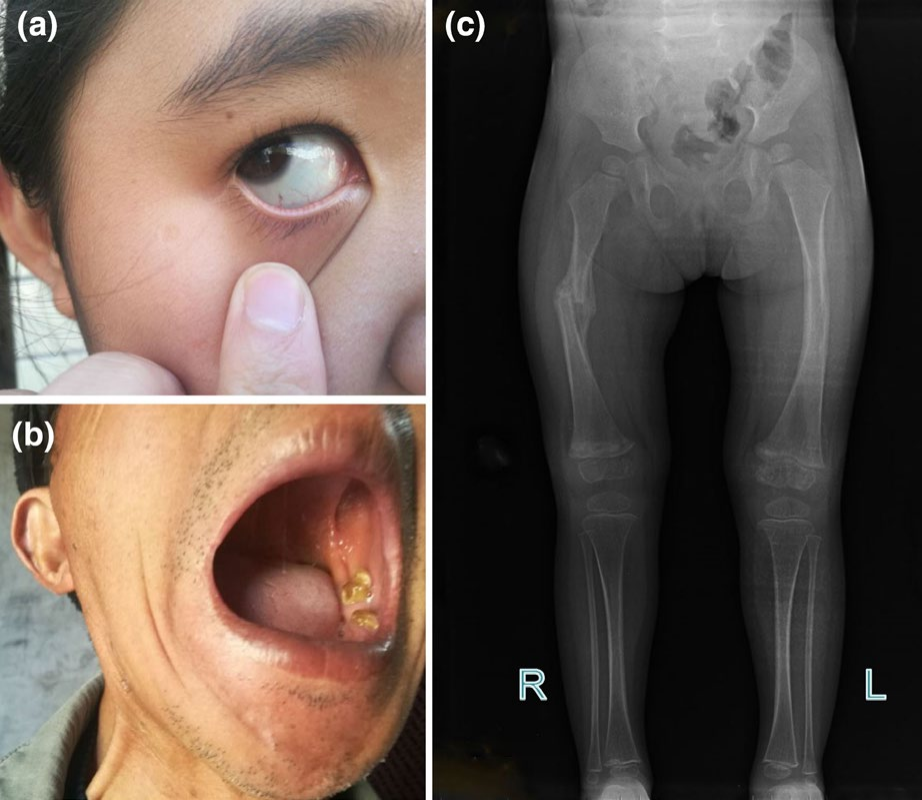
(a) The proband (IV:1) presents with blue sclera. (b) Clinical picture shows dentinogenesis imperfecta in patient (II:2). (c) Radiograph shows fractures and abnormal callus formation of the proband (IV:1) resulting in slight deformations of long bones
